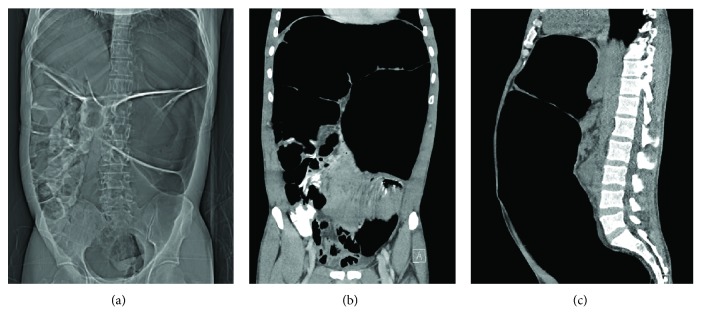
Preoperative computed tomography of the abdomen. (a) The initial scout film shows severely dilated sigmoid colon. (b) Coronal section. (c) Sagittal section.
